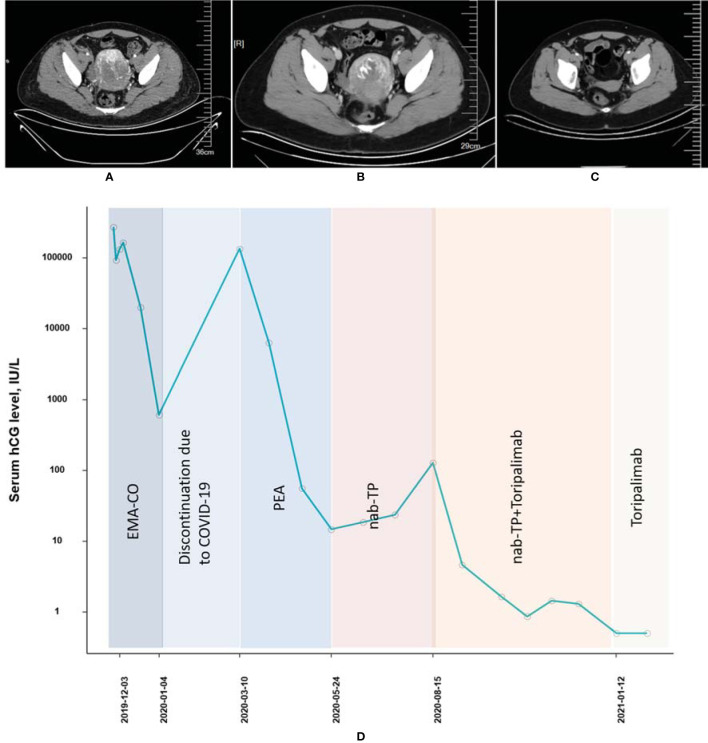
Computed tomography imaging (A) before EMA-CO chemotherapy, (B) after EMA-CO chemotherapy, and (C) after combined treatment of PD-1 and chemotherapies (patient 2). (D) The concentration of the β-hcg level since the diagnosis of choriocarcinoma (patient 2).
